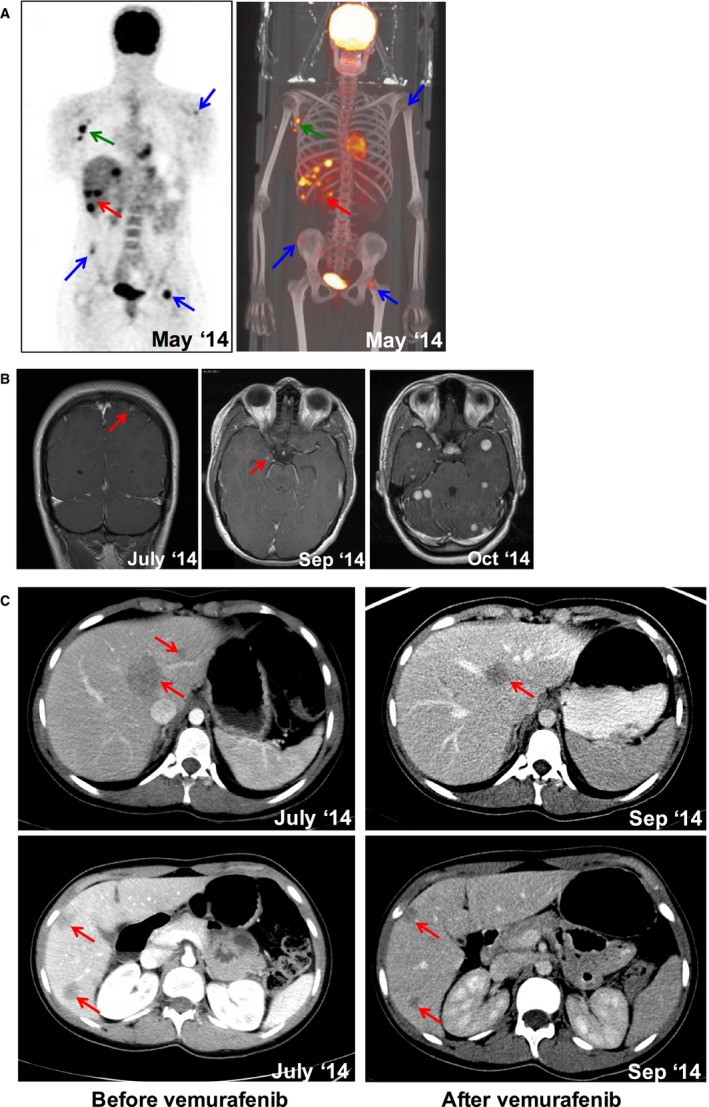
Radiological evaluation through treatment. (A) 18F‐FDG PET/CT alongside 3D colour reconstruction. Arrows indicate avid FDG tracer uptake in the right axilla, left humeral head, left femoral neck and right iliac crest (blue) as well as widespread liver uptake (red). (B) Post‐contrast T1‐weighted MR images showing tiny enhancing lesions in the left parietal lobe (July 2014) and right amygdala (September 2014). Axial post‐contrast MR images prior to whole‐brain radiotherapy showing multiple and supra‐ and infratentorial lesions with no significant mass effect (October 2014). (C) Cross‐sectional CT images of the liver post‐IV‐contrast in the portal phase. Baseline images show hypodense focal lesions corresponding to segment 1 in the left hepatic lobe (left upper) and the caudal segments of the right hepatic lobe (left lower). On the right, post‐treatment images indicate a partial response in all liver lesions (arrows).
